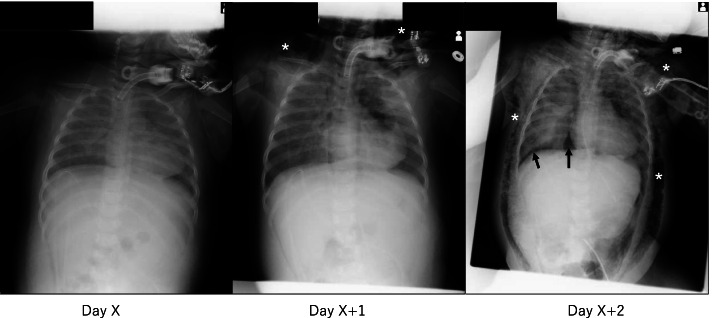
Representative X-ray images of a patient (an 8-month-old female with weight 5.7 kg and height 60 cm) [5]. On day X, good echocardiographic imaging was obtained; however, the image quality was slightly reduced than the day before. There was no remarkable change suggesting pneumatosis on the X-ray image. On day X + 1, good echocardiographic imaging was not obtained. Taking a closer look at the X-ray image, subcutaneous emphysema (asterisks) is observed around the neck. We overlooked this sign because we were distracted by the change of the X-ray image of the left lung field. On day X + 2, the patient developed severe pneumatosis. The right-side X-ray image shows extensive subcutaneous emphysema (asterisks) and pneumothorax (arrows)
